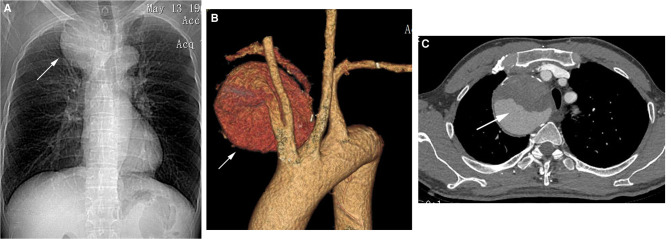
(A) Chest X-ray showed an intrathoracic mass in the upper lobe of the right lung and displacement of the trachea to the left (white arrow). (B,C) Computed tomography angiography (CTA) revealed a giant right subclavian artery aneurysm (SAA) with partial intraluminal thrombus and severe angulated aneurysm necks (white arrow).
